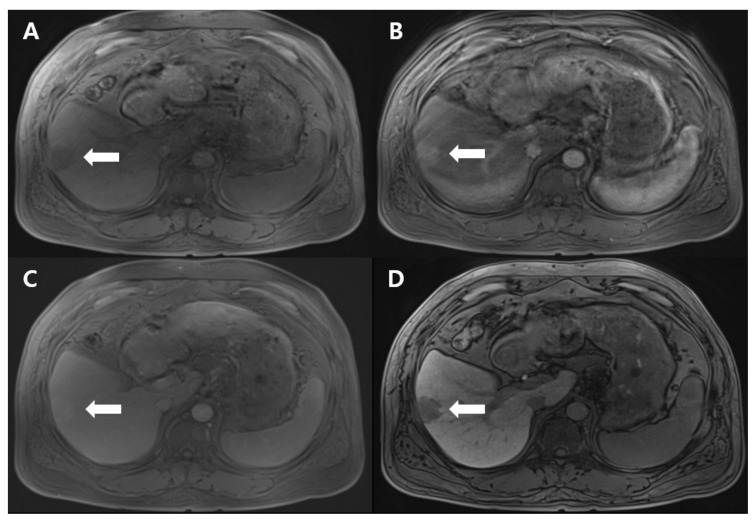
T1-weighted magnetic resonance imaging reveals an approximately 3-cm-sized, lobulated, hypointense mass in the pre-contrast phase (A), a hyperintense mass in the arterial phase (B), an isointense mass in the delayed phase (C), and a hypointense mass in the hepatobiliary phase (D), at segment 6. The lesion is indicated by white arrows in all phases.
